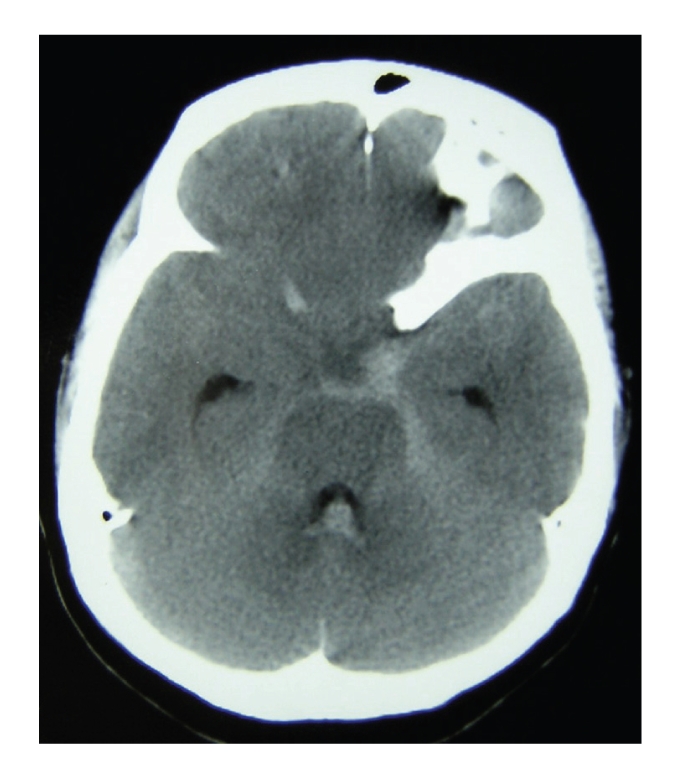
A head CT scan of the presented case. A head CT scan showed subarachnoid hemorrhage in the supracella cistern with hematoma in the fourth ventricle. Cerebral edema with sulcal effacement is also recognized.
